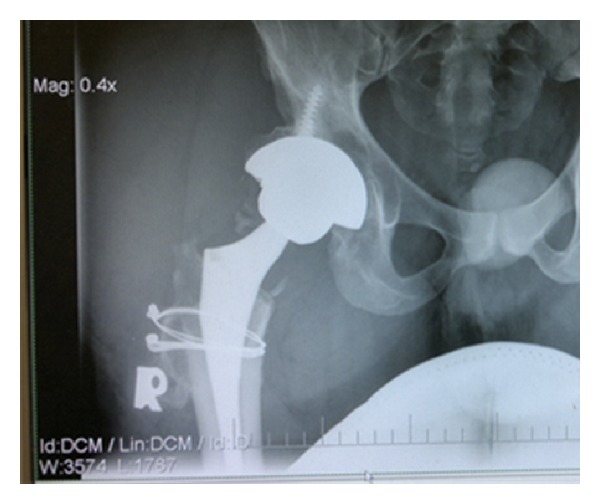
The anteroposterior radiograph of the right hip at 1 year postoperative: showing relative lateral position of the socket to improve offset and 4-5 cm distal advancement of the greater trochanter.
